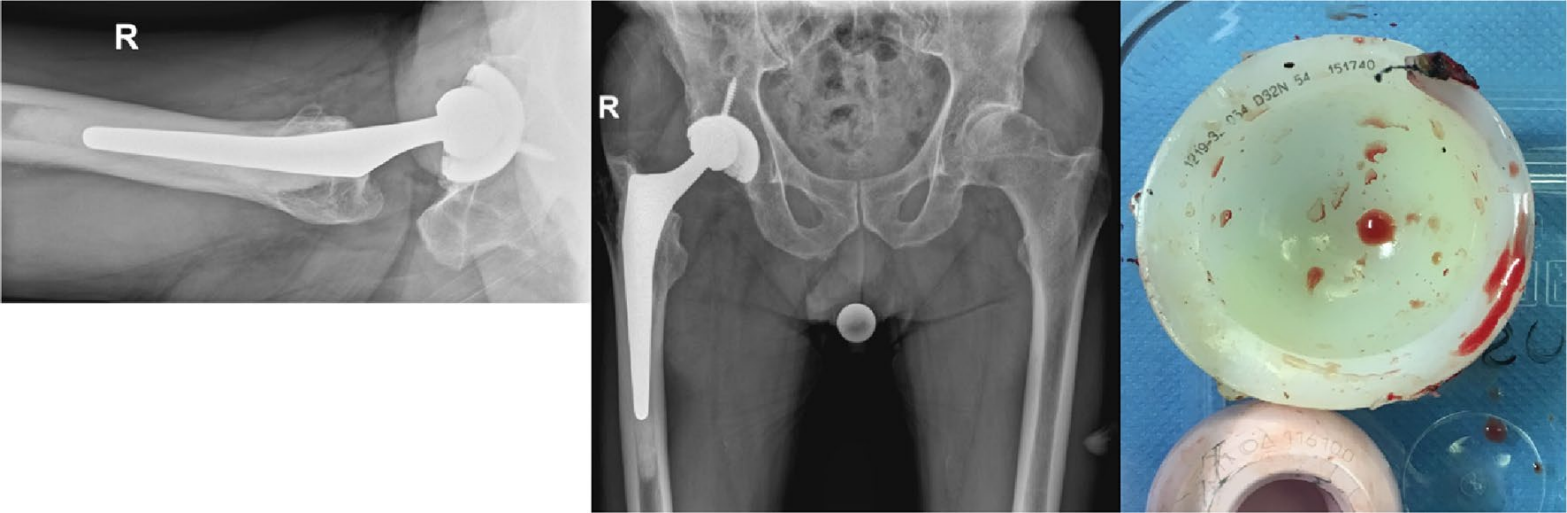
Example of dissociated Pinnacle liner from a patient we had treated (left axial hip X-ray; center low ap pelvis X-ray; right photograph after removal of liner with four damaged or missing anti-rotation tabs at time of removal)
